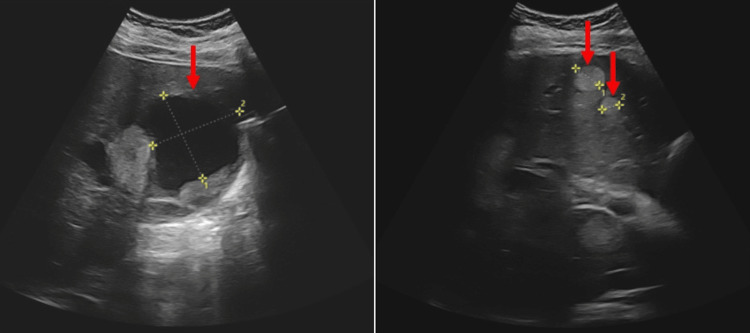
Abdominal ultrasound showed several cystic lesions and multiple mass lesions in the liver (red arrows).
